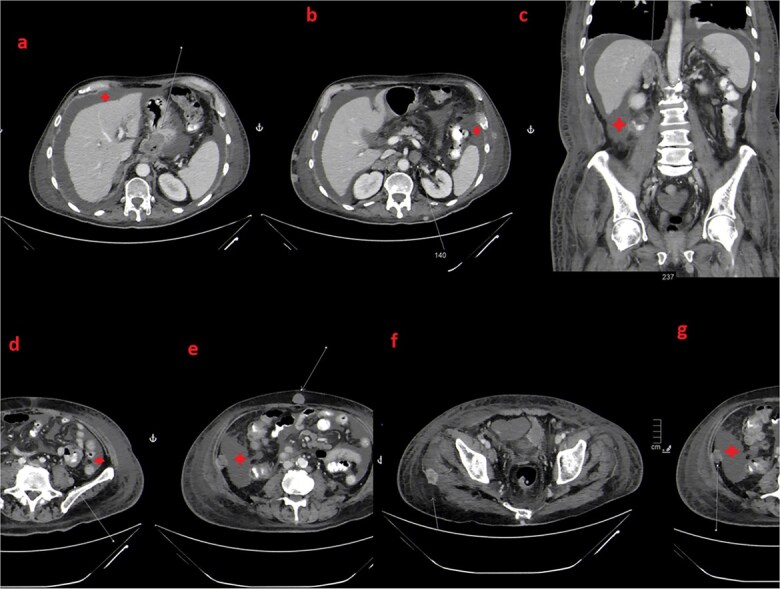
Axial (a, b, d, e, f and g) and Coronal (b) images from follow up CT scan after 4 months show progression of the GE junction primary tumor (a), right adrenal metastasis (c) and many preexisting subcutaneous and muscular deposits (e). Also, there is the development of new ascites (star), peritoneal deposits (g) and other muscular and subcutaneous lesions (d and f).
